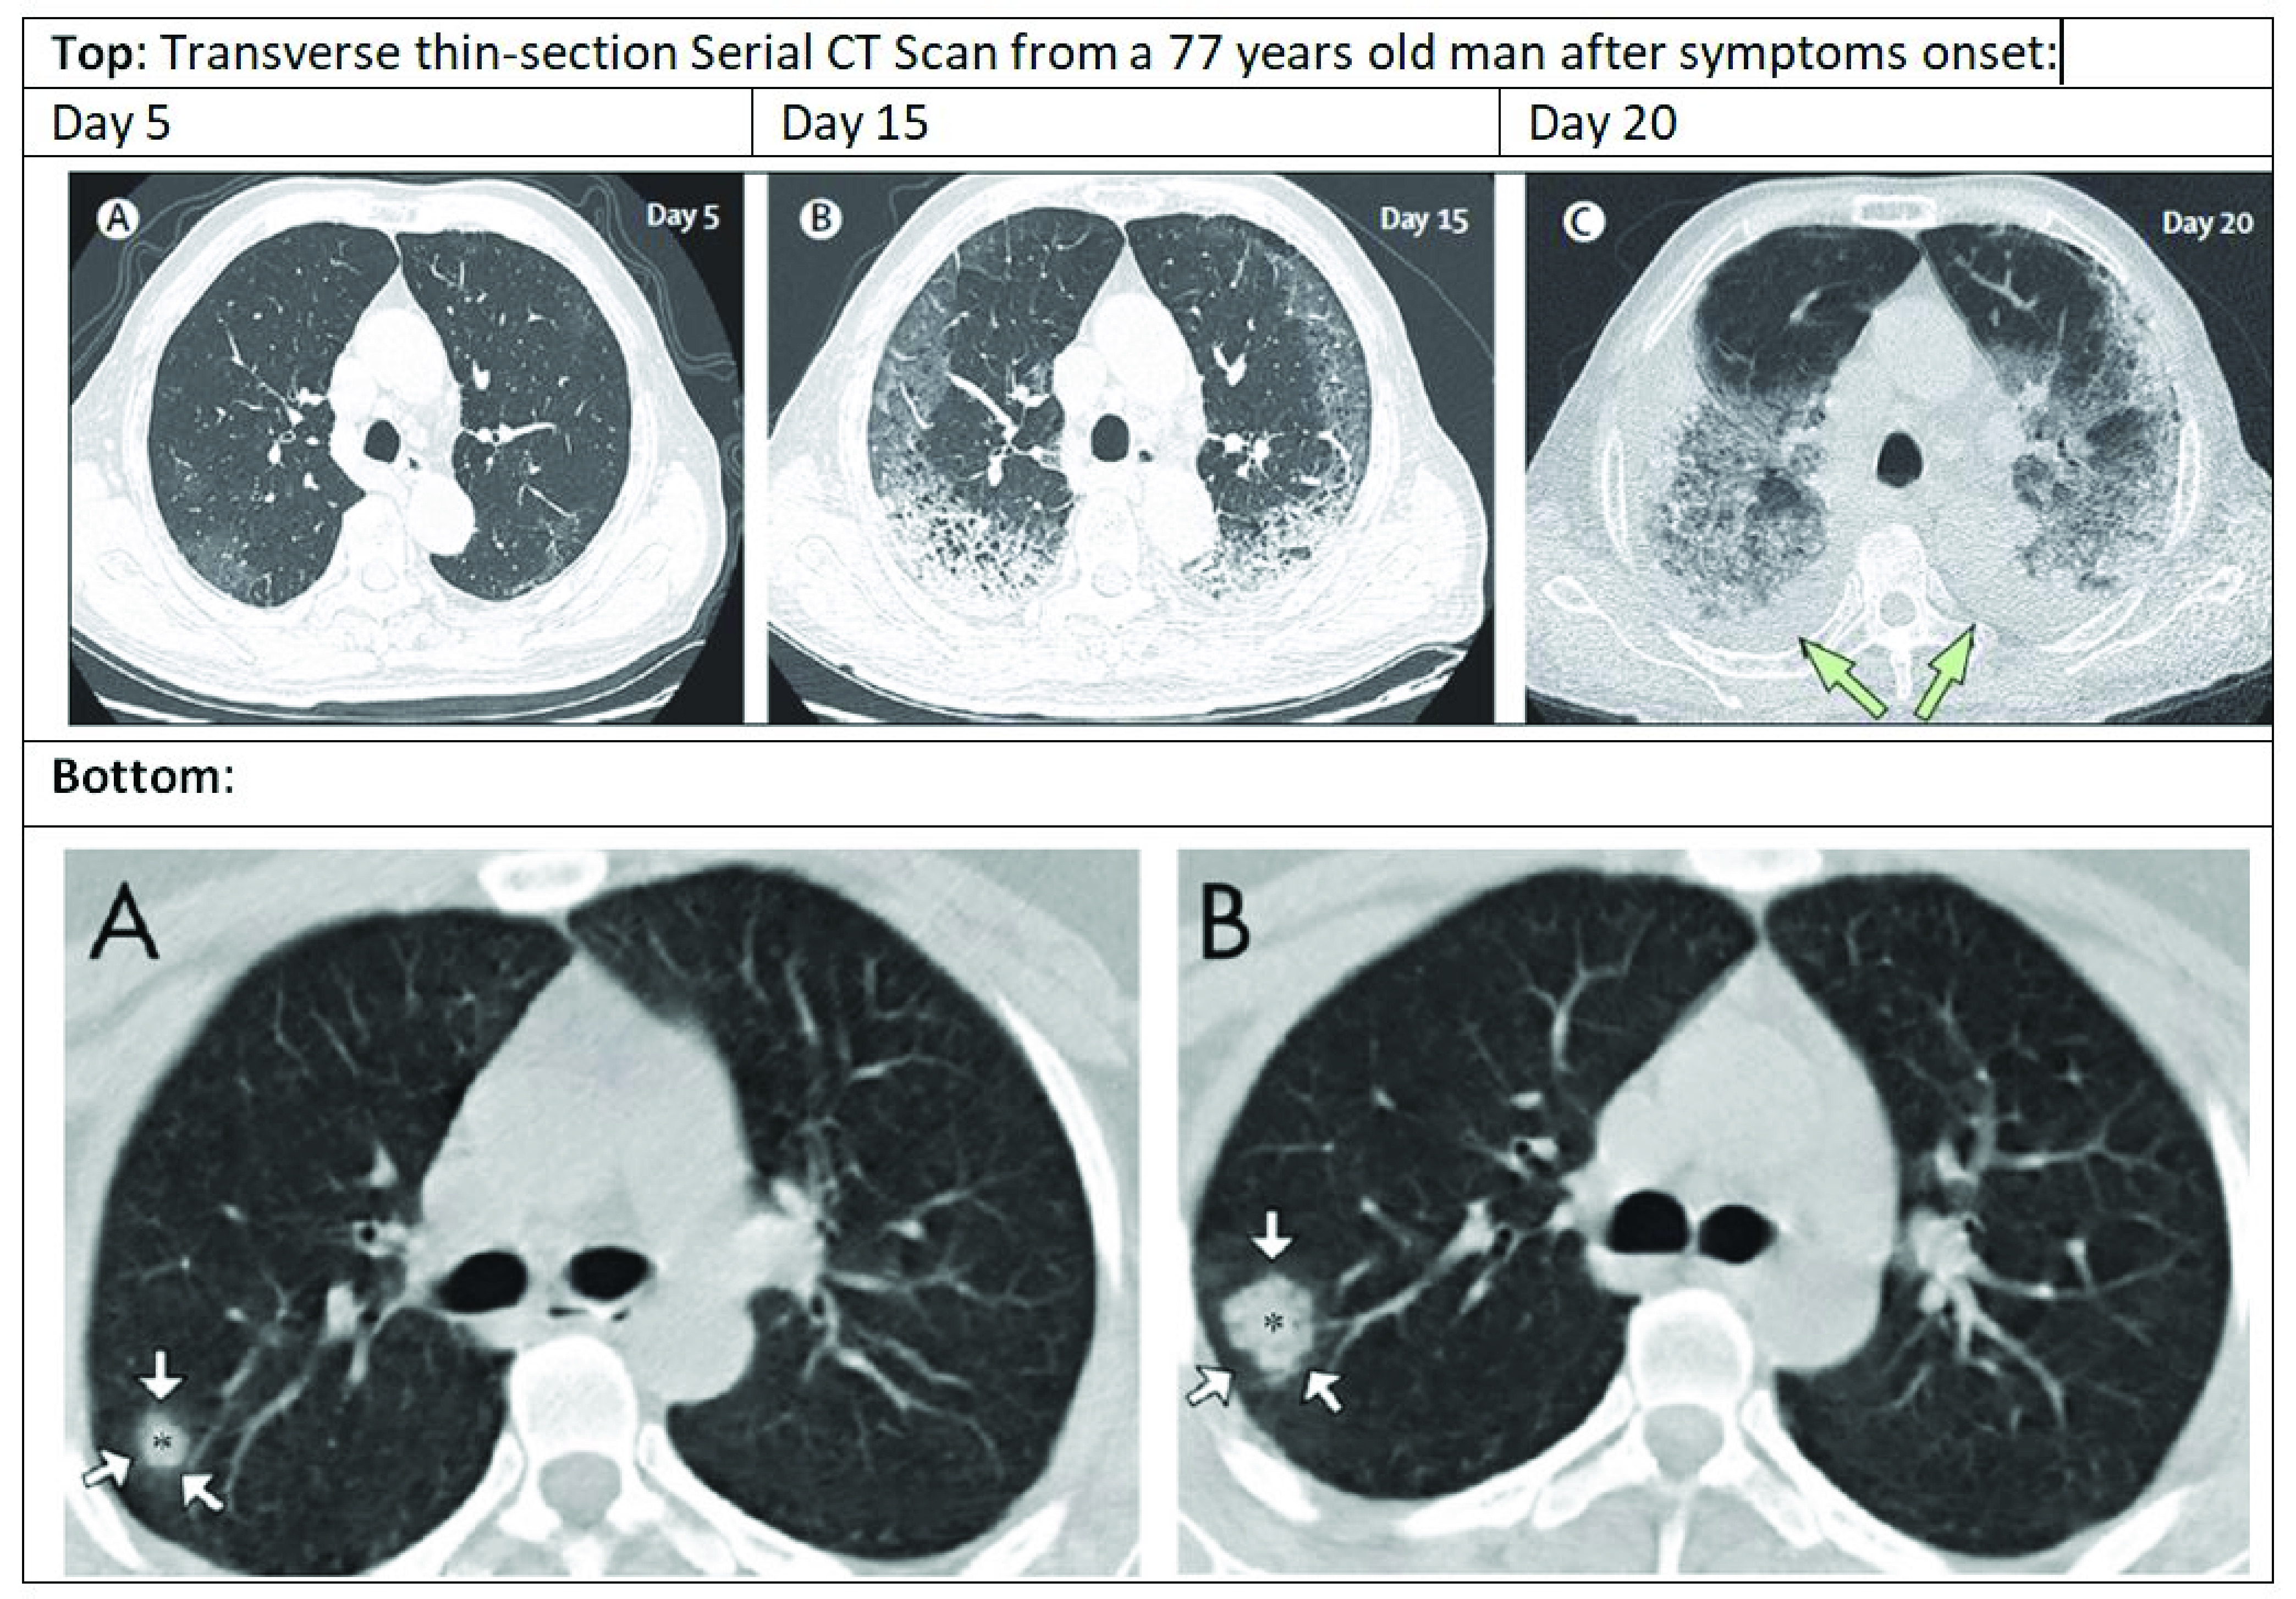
CT images adapted from [6], [18] portray CT features related to COVID-19. Ground glass opacities (top) and ground glass halo (bottom).
